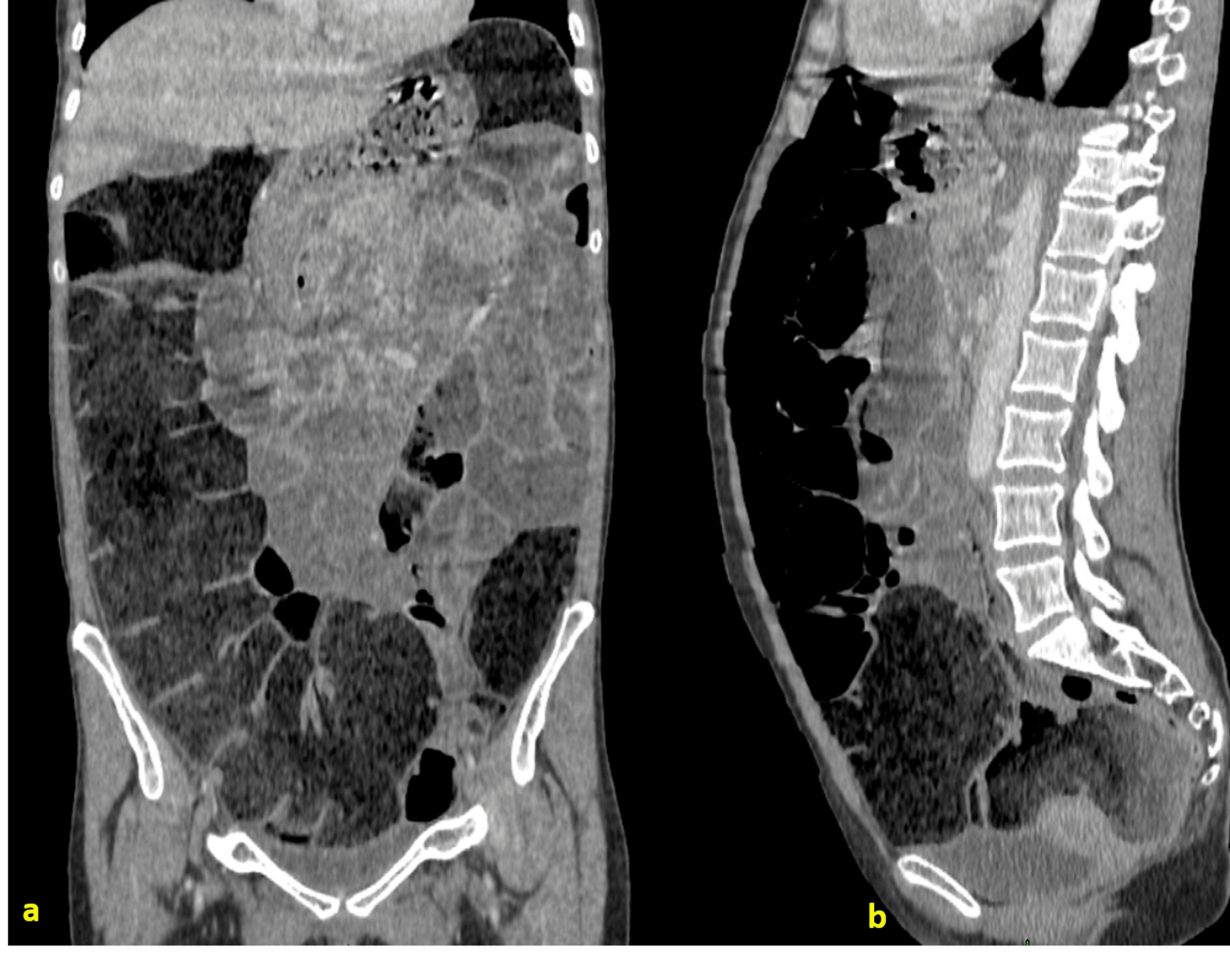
Contrast-enhanced abdominal CT, coronal and axial views, showing diffuse colonic and small-bowel distension without any identifiable transition point a: Coronal view
